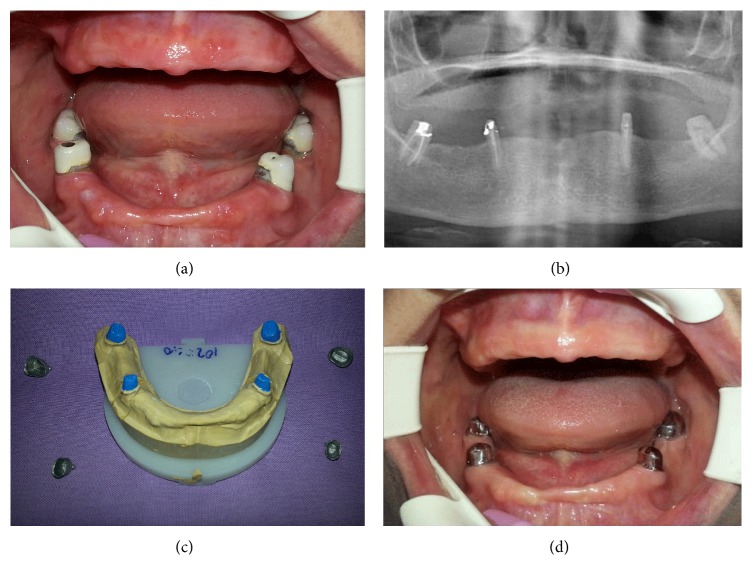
(a) Frontal and (b) orthopantomographic view of the case before treatment. The image of the primer copings on (c) cast model (d) and intraoral examination.
